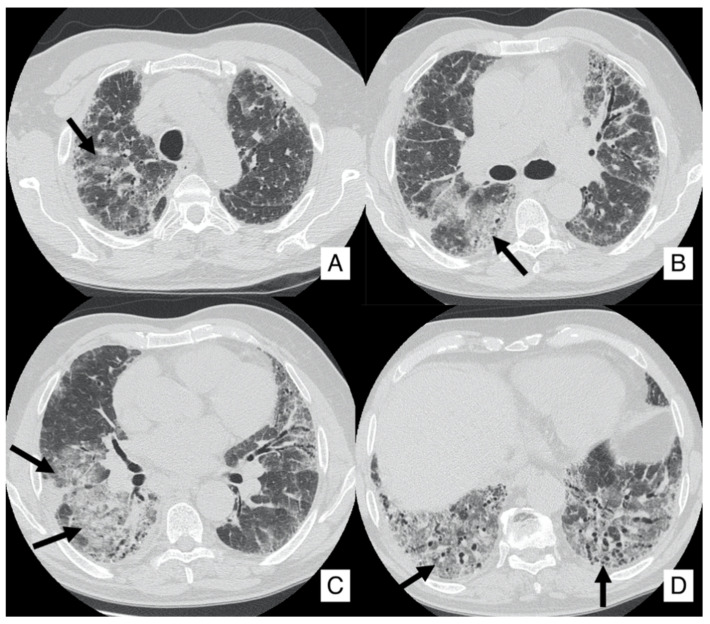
Acute exacerbation of IPF in an 80-year-old man (A–C). These CT scans demonstrate an increased diffuse ground-glass opacity with an increased parenchymal density, especially in the right upper lobe (black arrows in A and B) and in the upper part of the right inferior lobe (black arrows in C). At the pulmonary bases, traction bronchiectasis is clearly recognized (black arrows in D). This increased GGO pattern is related to an acute exacerbation of IPF. At clinical evaluation, a decreased vesicular murmur and wheezes in the basal-middle areas were found.
